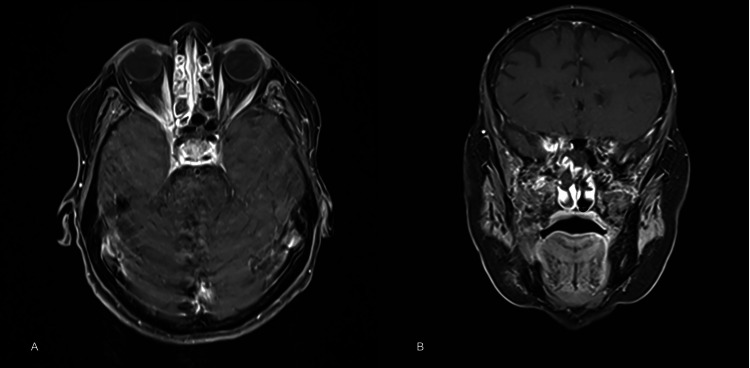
MRI findings A: MRI head and orbits revealing relatively diffuse soft tissue thickening and enhancement at right orbital apex in axial planes; B: coronal planes. The right optic nerve sheath is mildly enhancing compared to the left, with a normal appearance in signal and caliber of the optic nerve itself.
